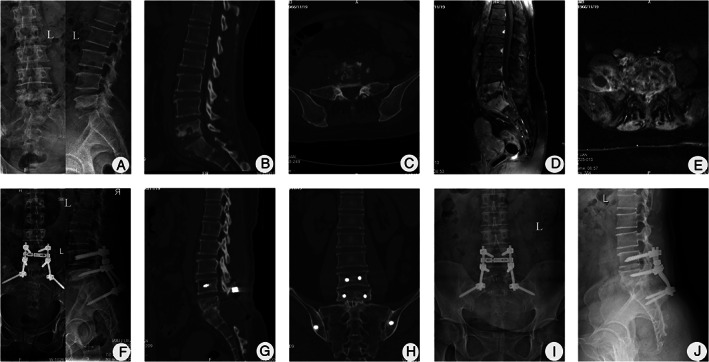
A 52‐year‐old female with L_5_/S_1_ TB underwent one‐stage posterior debridement, interbody fusion, and lumbopelvic fixation. (A–E) Preoperative images showing that the TB lesion was located at L_5_/S_1_ and the lesion involved vertebral body destruction (the pedicle of S_1_ was also affected) and abscess formation. The lumbosacral angle was 23°. (F) Postoperative radiograph depicting correction of the deformity and the lumbosacral angle at 28°. (G, H) CT showing solid bone fusion was achieved at 15 months. (I, J) X‐ray displaying the instruments in the correct position, with a lumbosacral angle of 27° at the follow‐up period of 60 months.
